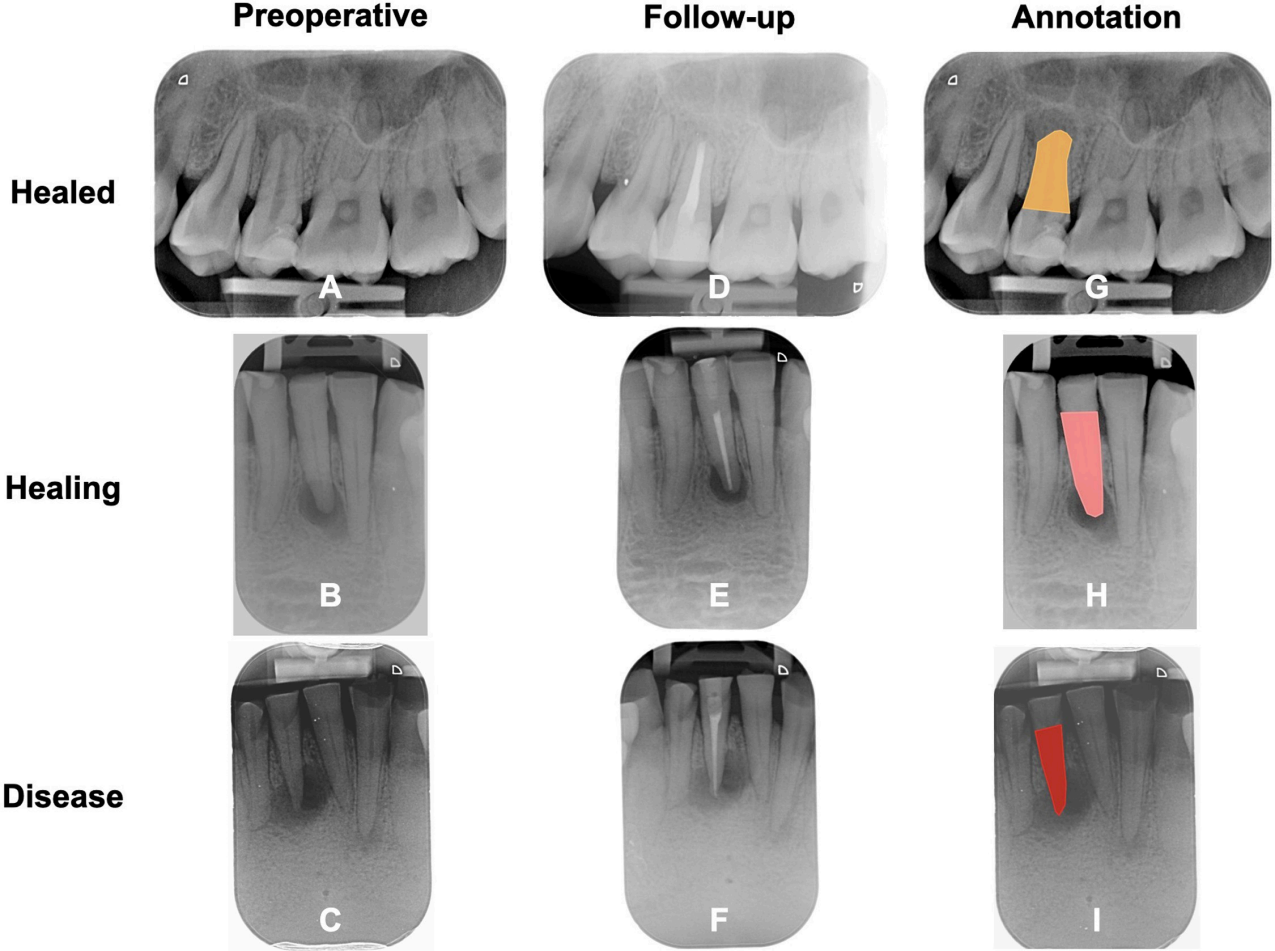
Examples of preoperative radiographic images (A-C); follow-up postoperative radiographic images (D-F); and polygon annotation on the preoperative radiographic images (G-I) of healed, healing and disease cases.
